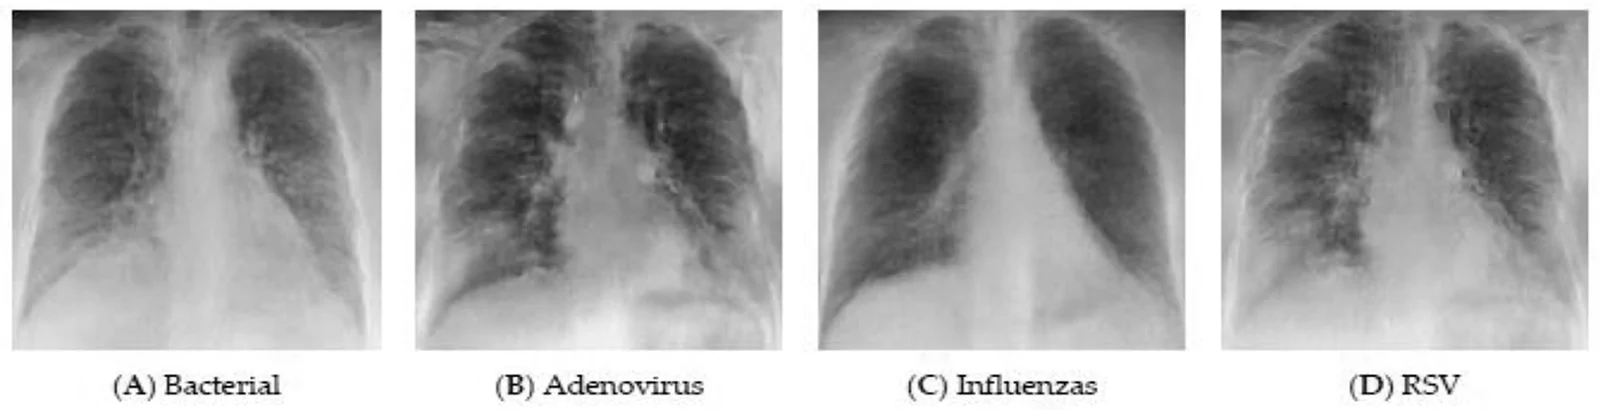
Representative chest X-ray examples highlighting radiographic manifestations of respiratory infections: (A) Bacterial pneumonia, (B) Adenovirus, (C) Influenza, and (D) Respiratory Syncytial Virus (RSV). The images demonstrate differences in lung opacity patterns associated with each condition [14]. Reproduced from “Samples of synthetic CXR images” by Albatoul S. Althenayan, Shada A. AlSalamah, Sherin Aly, Thamer Nouh, Bassam Mahboub, Laila Salameh, Metab Alkubeyyer and Abdulrahman Mirza, licensed under CC BY.
